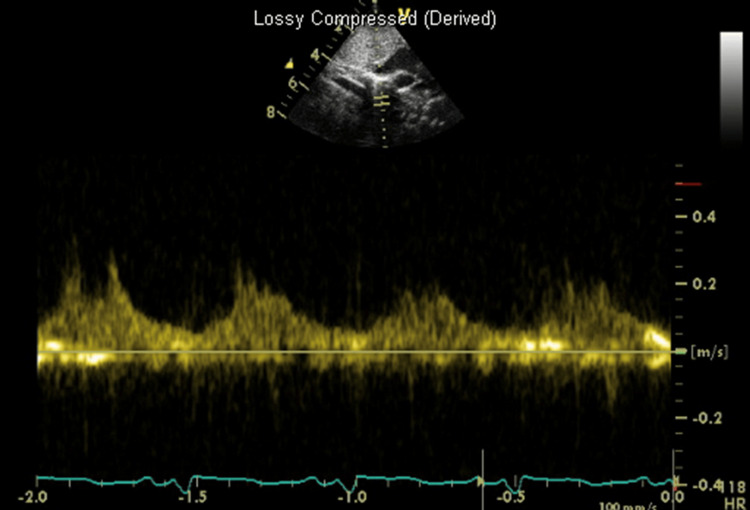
Coarctation of the aorta Subcostal view in transthoracic echocardiography showing reduced flow velocity at the abdominal aorta level with a diastolic “tail” consistent with severe coarctation of aorta with reduced pulse width.
